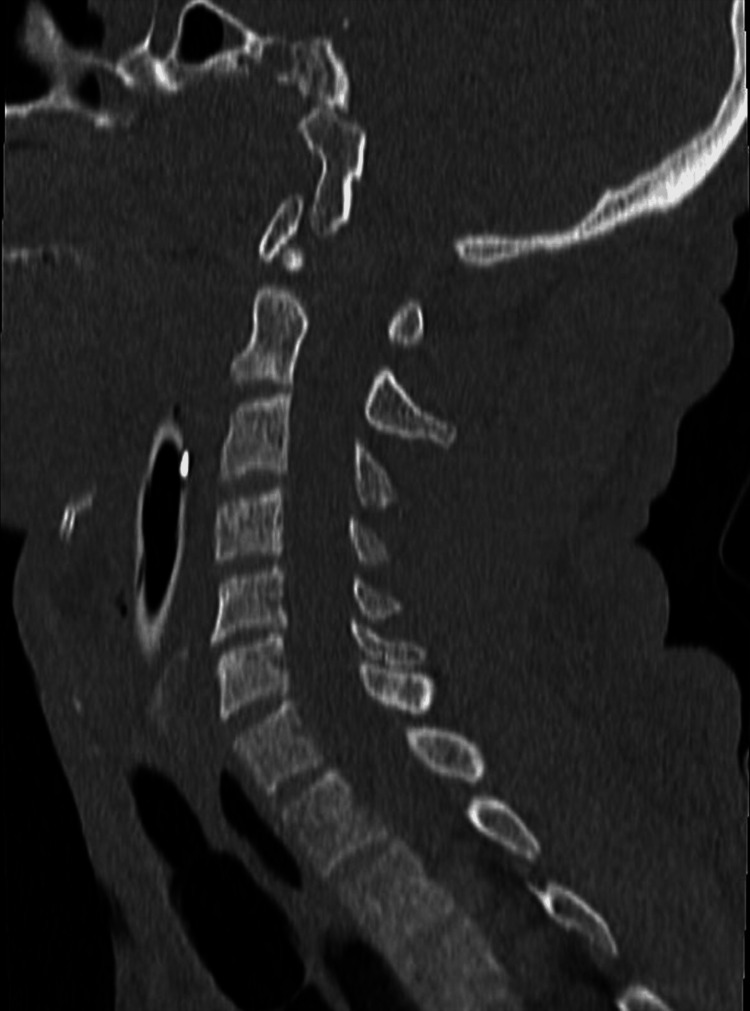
Neck CT scan of the first patient (FR) This sagittal CT image of the cervical spine demonstrates a normal osseous anatomy. The vertebral bodies are well-aligned, with preserved vertebral height and intact cortical margins, showing no signs of compression fractures or lytic lesions. The intervertebral disc spaces are maintained throughout, suggesting the absence of significant degenerative disc disease. The spinous processes, laminae, and pedicles appear intact and symmetric. CT: computed tomography
